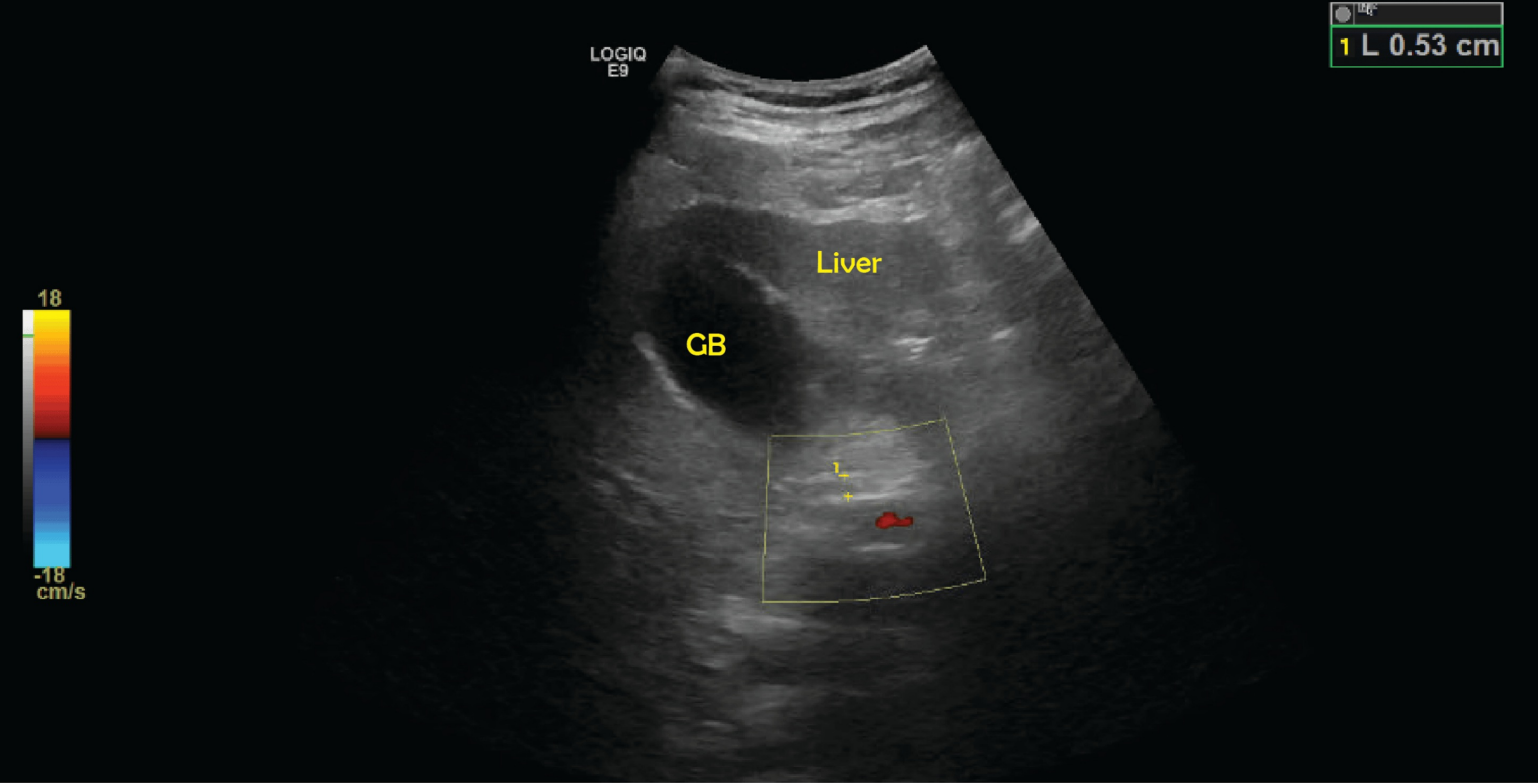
Ultrasound of the abdomen showing normal common bile duct (5.3 mm)
